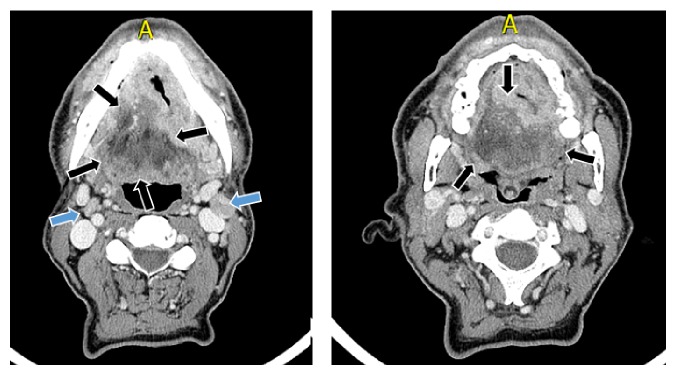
Computed tomography (CT) finding of case one showed that a heterogeneous mass can be seen which spreads to the back of the tongue with extension to the floor of the mouth without reaching the sublingual space (black arrows). The CT confirmed the existence of metastatic lymph nodes (blue arrows).
